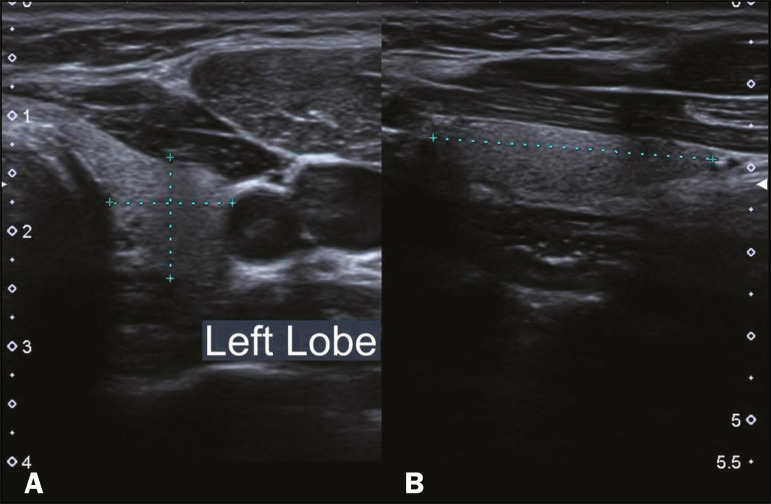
Thyroid ultrasound examination of a euthyroid child. The left thyroid lobe (between the markers) is observed in the transverse section for laterolateral measurement (A) and in the longitudinal section for craniocaudal measurement (B).
